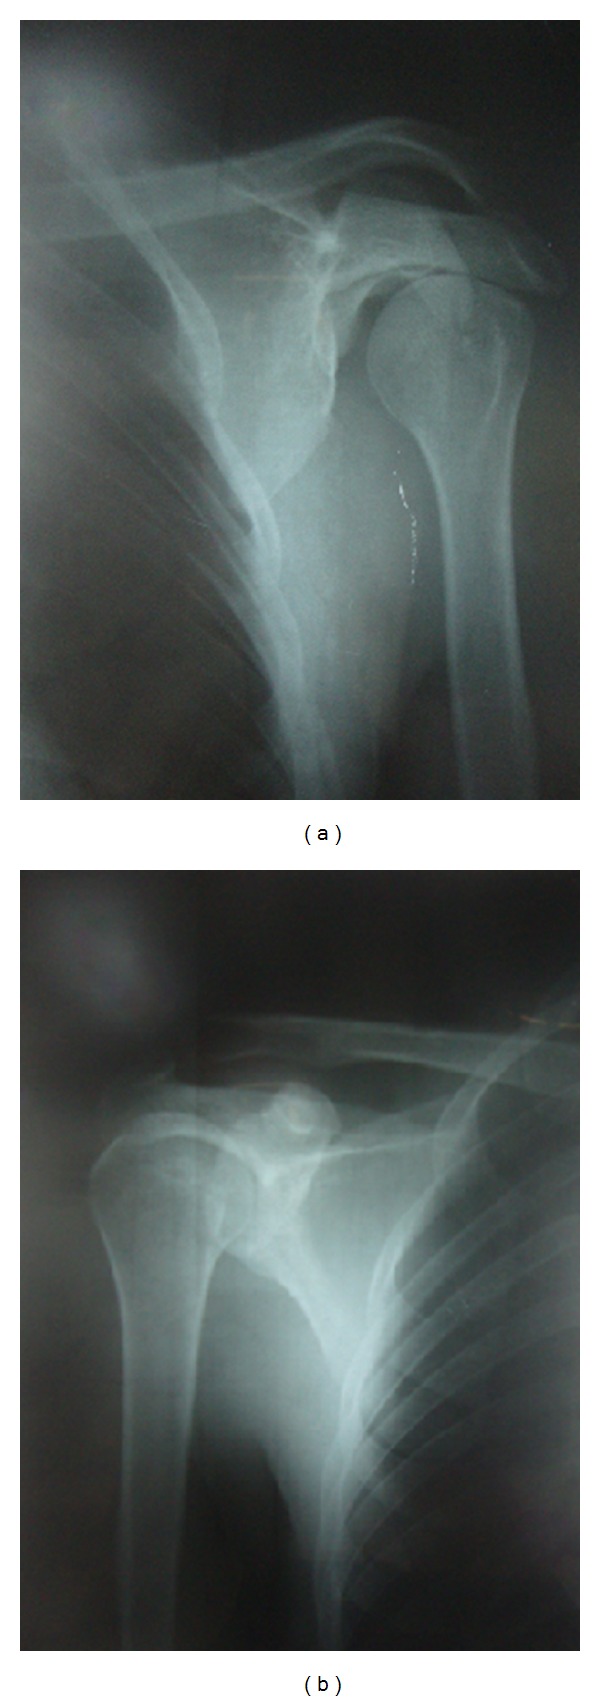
(a) shows Left-sided glenoid hypoplasia with normal humeral head. (b) shows normal right-sided shoulder radiograph.
